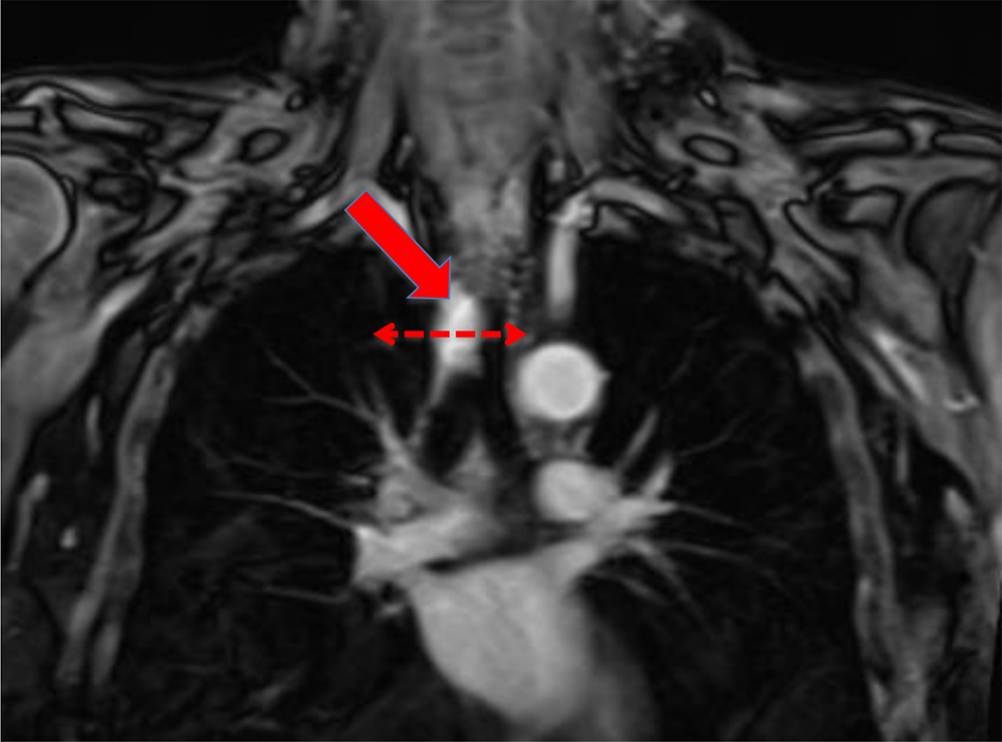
Contrast-enhanced MRI of the mediastinum: coronal slice. Block arrow: recurrent tumour, pre-treatment (November 2011). Broken arrow: level of previous surgical resection and end-to-end anastomosis.
